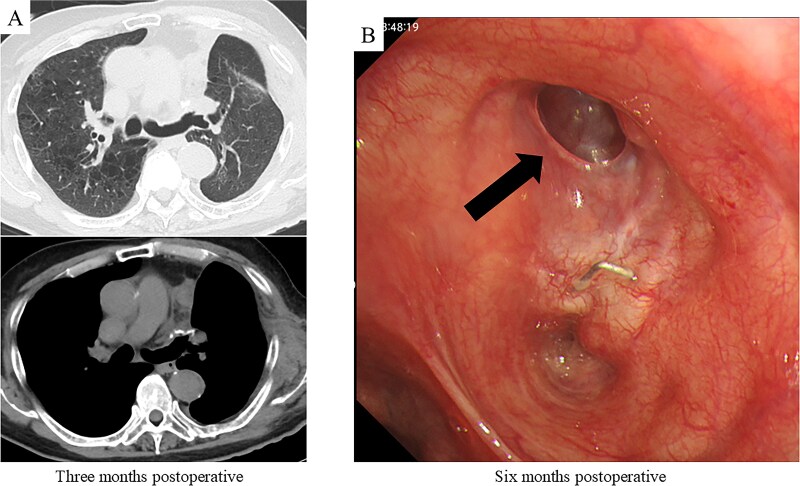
Postoperative CT imaging and bronchoscopic evaluation. (A) CT scans at 3 months postoperatively reveal no recurrence of air leakage, indicating a stable clinical course. (B) Bronchoscopic examination at 6 months postoperatively shows satisfactory granulation tissue formation at the upper lobe bronchial stump, consistent with complete healing.
